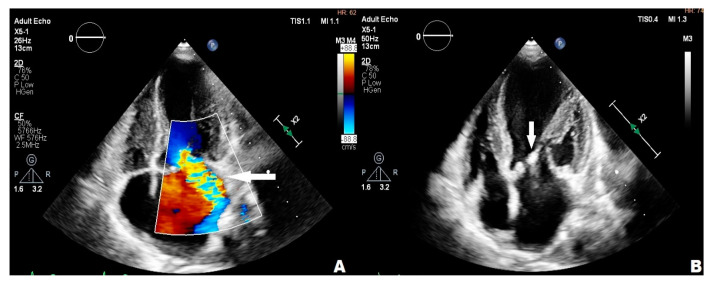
Transthoracic echocardiography; (A) arrow point at the mitral regurgitation wave; (B) arrow point at hyperechogenic 4 mm × 7 mm lesion in mitral chordae.
